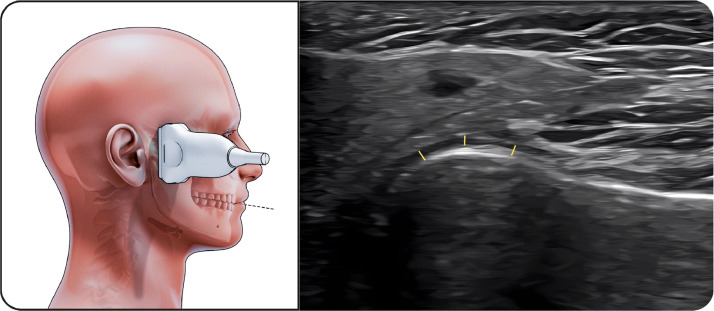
Lateral capsule-condyle distances. The lateral capsule-condyle distance is defined as the shortest linear distance between the hyperechoic joint capsule and the cortical surface of the mandibular condyle, assessed using a longitudinal ultrasonographic scan. On the ultrasound image, the mandibular condyle is identified by its hyperechoic cortical surface with posterior acoustic shadowing, while the joint capsule appears as a hyperechoic linear structure superficial to the condyle. The parotid gland is visible superficial and lateral to the temporomandibular joint as a heterogeneous echogenic structure, and the parotid duct (Stensen’s duct) may be identified as a tubular, hypoechoic structure within the gland. These anatomical structures serve as reference landmarks during measurement of the lateral capsule-condyle distance.
